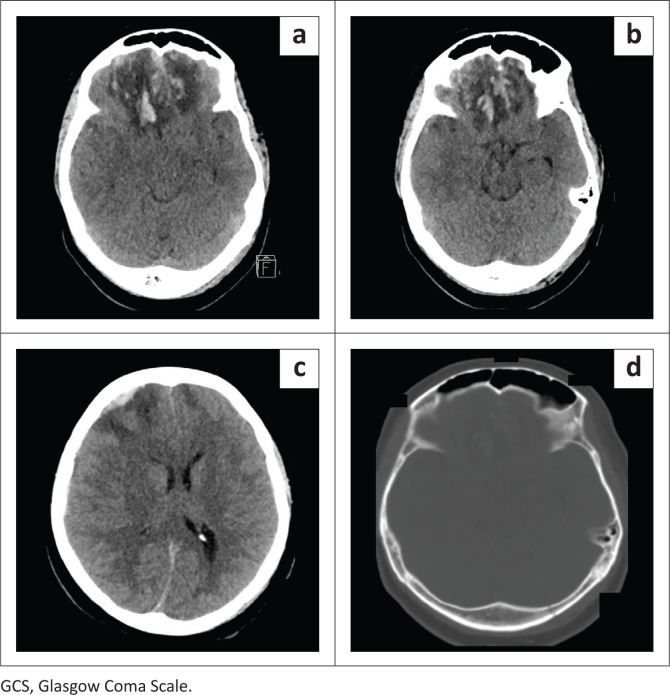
Thirty three-year-old female patient with history of fall from a moving vehicle presented with a Glasgow Coma Scale score of 13. Axial CT brain images on brain (a, b, c) and bone window setting (d) demonstrating bifrontal intraparenchymal and subarachnoid haemorrhages, perilesional oedema, frontal interhemispheric subdural haemorrhages (a), effacement of the basal cisterns (b), right frontal epidural haemorrhage, midline shift (c) and left occipital linear fracture (d).
